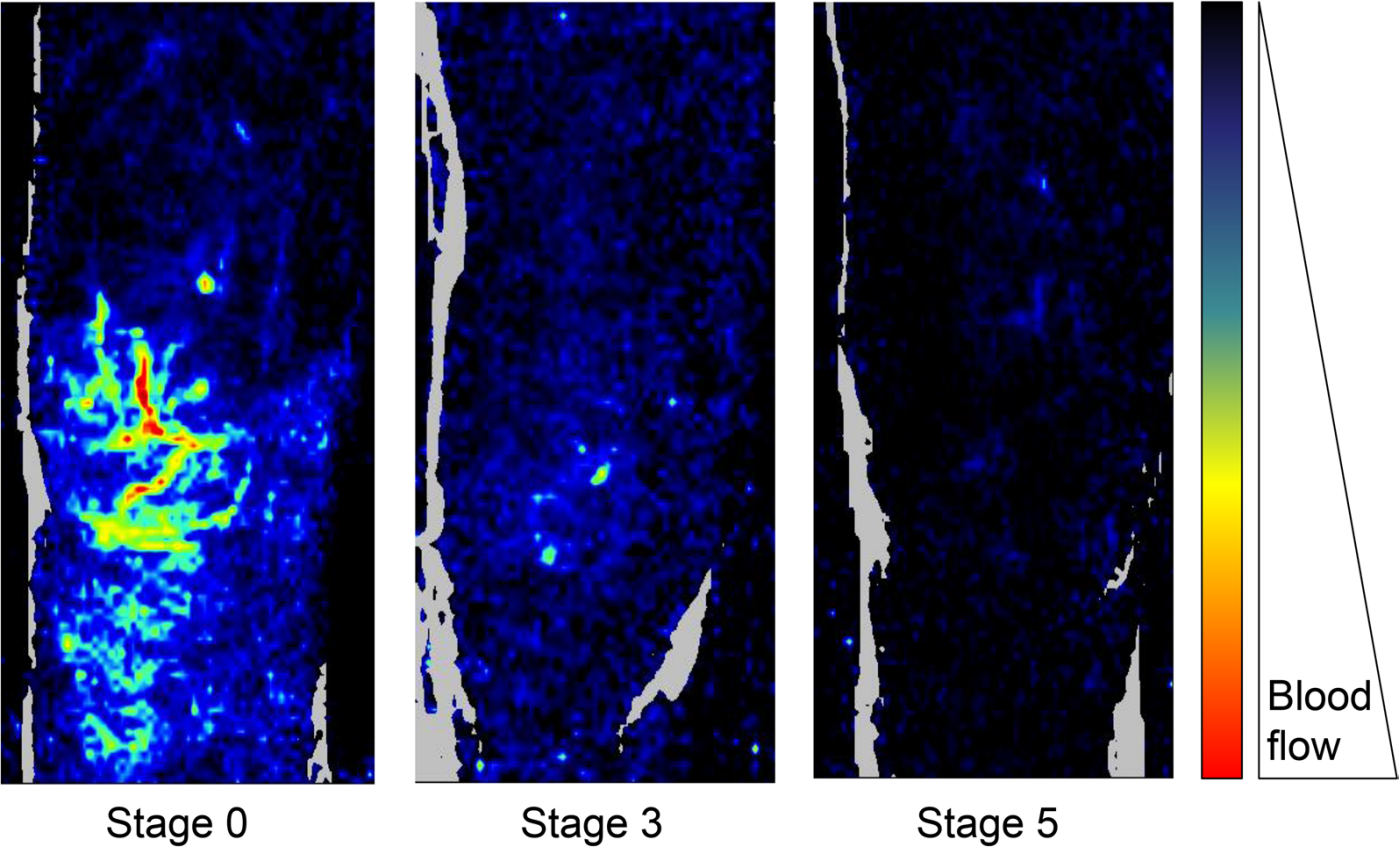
Examples of skin microvascular perfusion evaluation using laser Doppler imaging in the knee area according to the mottling score. Skin perfusion decreases when mottling score worsens. Adapted from [9]
